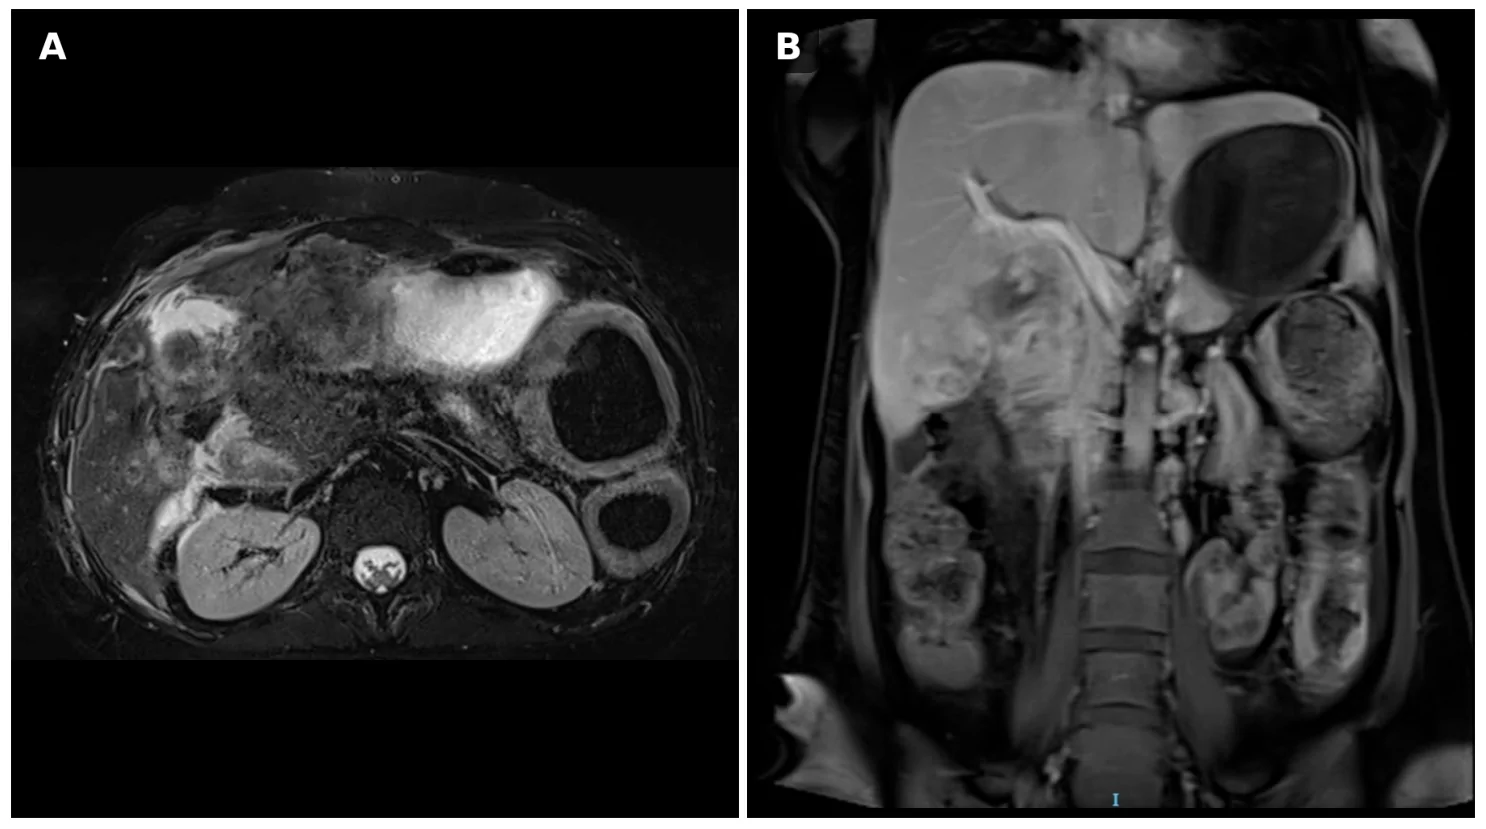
Contrast-enhanced MRI of the abdomen. (A) Axial image showing persistent inflammatory change at the gallbladder bed after cholecystectomy, extending into the adjacent hepatic parenchyma and the thickened gastric antrum; (B) coronal image showing periportal extension, skip-pattern bowel-wall thickening and a focal right renal lesion.
